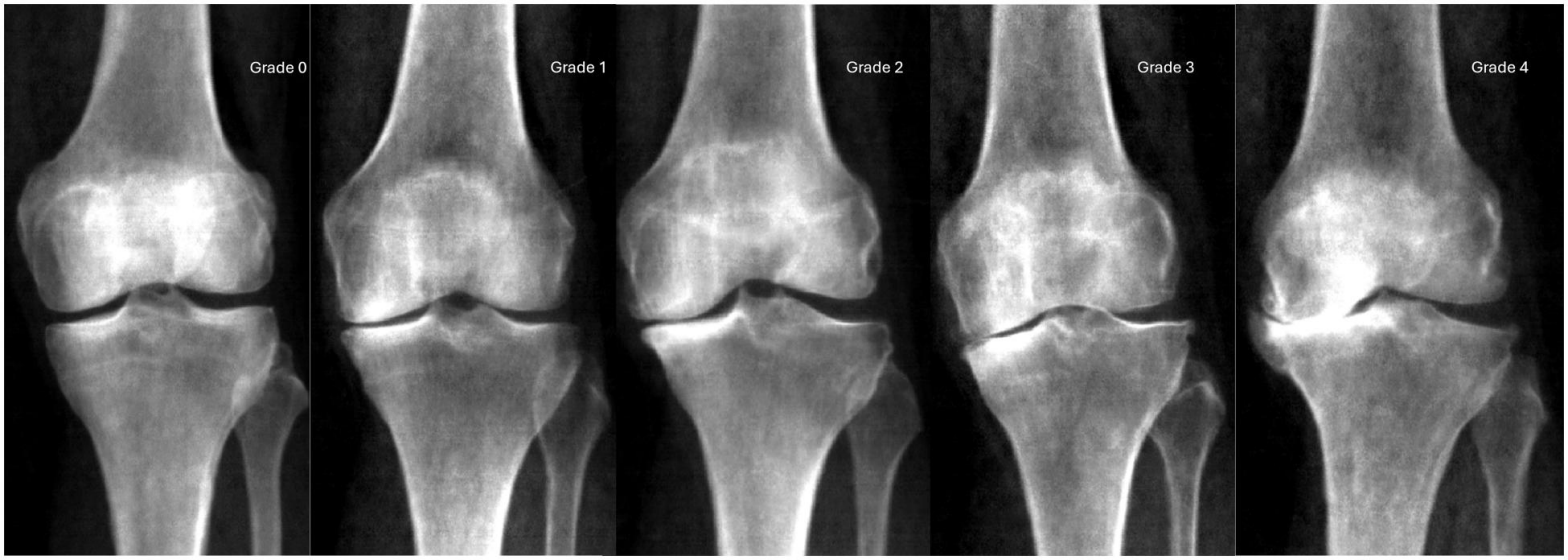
Example DXA scans representing each grade of rKOA. rKOA grades were generated by integrating data on osteophyte grades and JSN grades. The images show progression from grade 0 to grade 4, demonstrating increasing severity of OA changes. Grade 0 indicates no radiographic features of OA, while grades 1–4 show progressively more significant JSN and osteophyte formation
